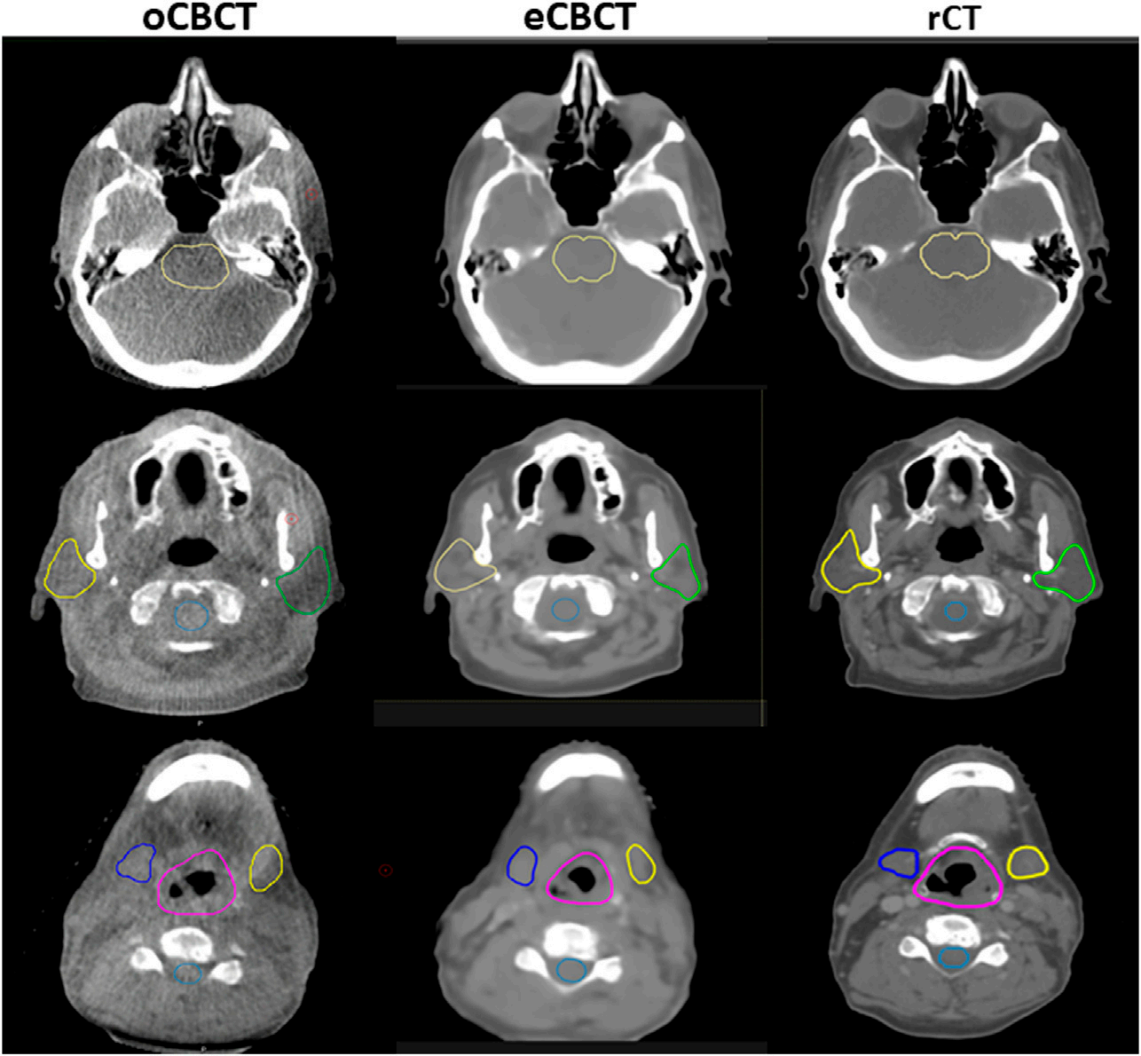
OARs delineated on transverse slices of oCBCT, eCBCT and rCT images for a representative HNC patient. OARs are outlined: brainstem (top, yellow line), parotids [middle, yellow (right) and green (left) lines], spinal cord (middle, light blue line),submandibular glands [bottom, blue (right) and yellow (left) lines], larynx (bottom, purple line).
